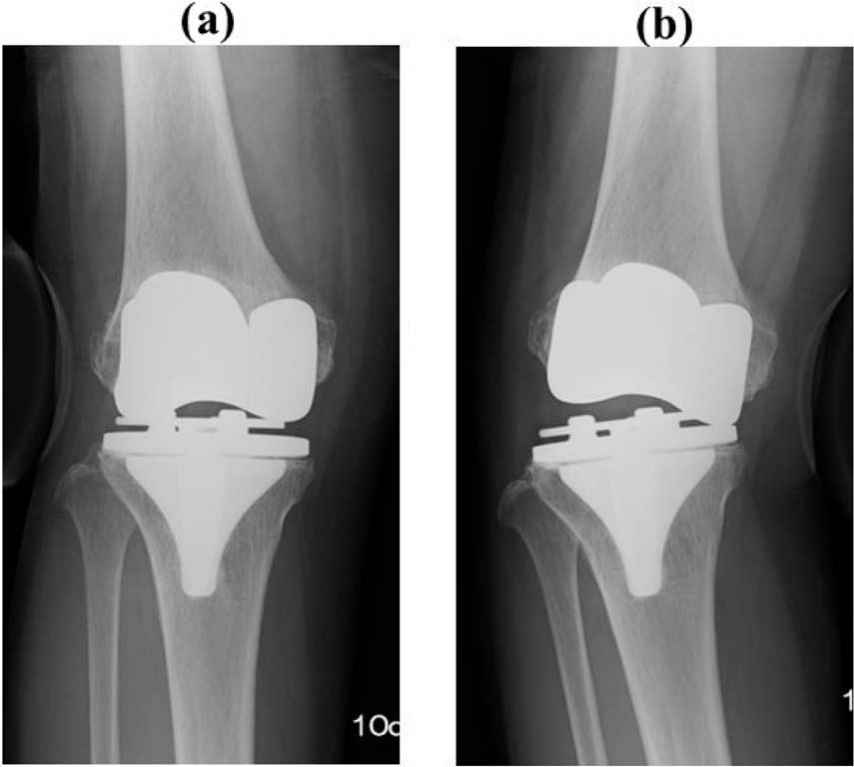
Post-operative varus and valgus stability of the knee joint in extension were assessed with stress radiography using a Telos arthrometer (Telos SD 900 Stress Device; Telos Medical Co., Ltd, USA). The patient was instructed to lie supine on a table for the measurement. During varus and valgus stress testing, a force of 150 N was applied just above the joint on the lateral or medial femoral condyle [8]. The patient had varus instability on the (a) valgus stress test and the (b) varus stress test.
